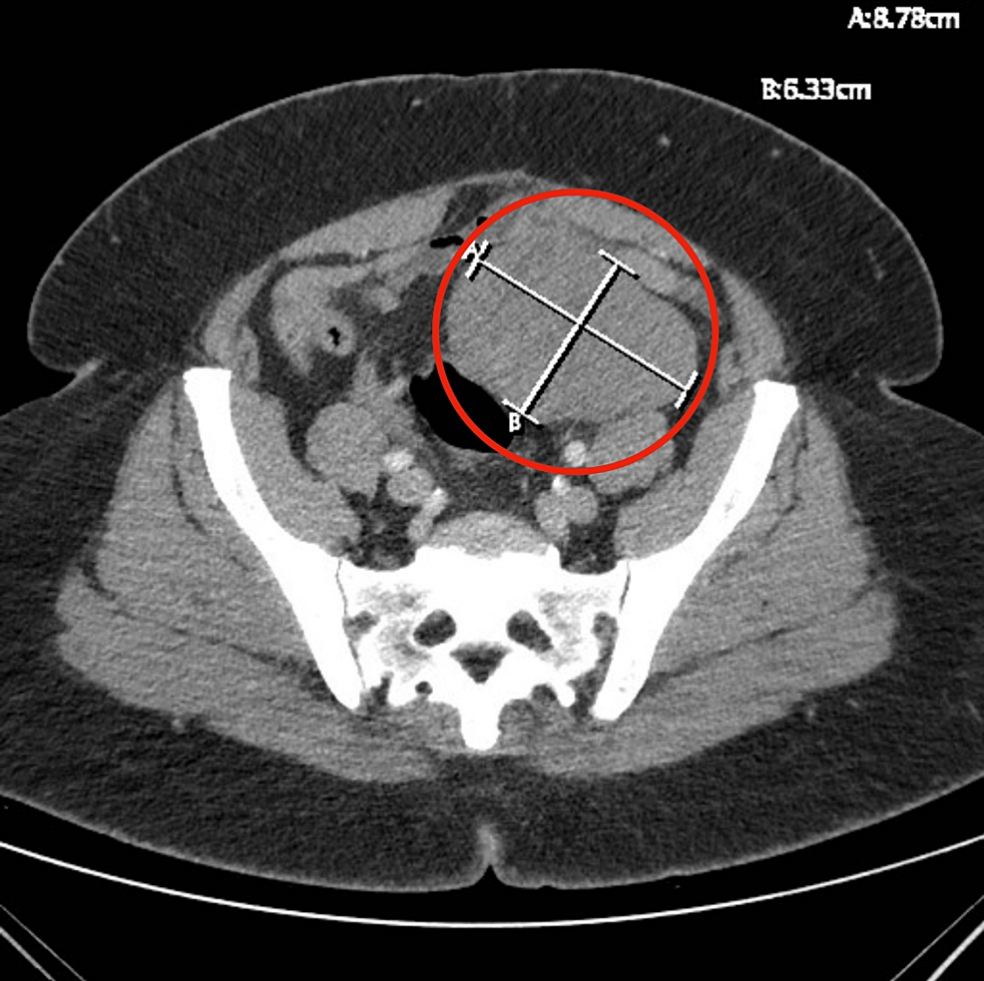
Computed tomography of the abdomen and pelvis showing a large mass in the left lower abdomen concerning for an ovarian mass (red circle)
